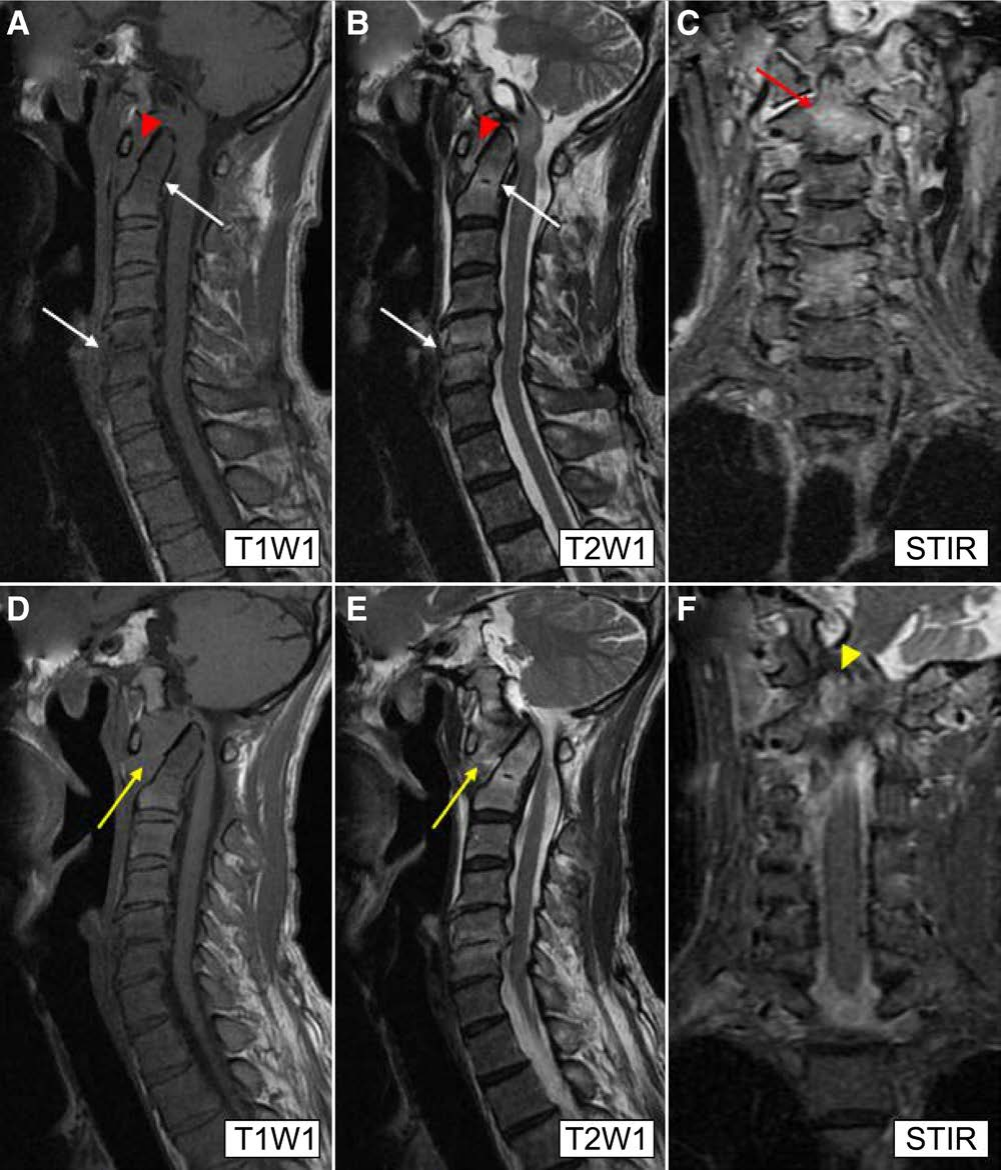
Radiological findings. (A–C) MRI on admission shows a lesion with low T1 (A) and high T2 intensity (B) at the C2, C5, and C6 vertebral bodies and the C5–C6 intervertebral disc in the sagittal section (white arrows). The coronal section (C) shows high-intensity with STIR at the C2 vertebral body (red arrow) in the coronal section. Additionally, atlantoaxial subluxation is suspected with the atlantodental interval of 5.27 mm (red arrowheads) (A, B). (D–F) The follow-up MRI of the sagittal section performed on day 31 reveals exacerbation of the atlantoaxial subluxation with an atlantodental interval of 10.02 mm (yellow arrows) (D, E). Imaging of the coronal section (F) reveals the expansion of a high-intensity area with STIR to the dens (yellow arrowhead). MRI = magnetic resonance imaging, STIR = short tau inversion recovery, T1WI = T1-weighted image, T2WI = T2-weighted image.
